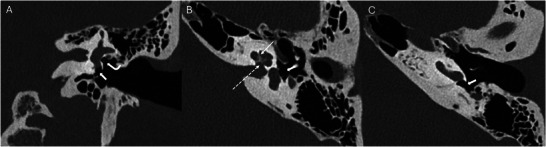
Photon‐counting detector computed tomography (PCD CT) images of the spiral structures within the human cochlea. Normal left temporal bone anatomic structures on PCD CT. A) Coronal PCD CT image demonstrating the position of the oval window (curved arrow) and round window (straight arrow). B) Axial PCD CT image at the level of the oval window (thick arrow) with insertion of the stapes crus. The modiolus (dashed arrow) and spiral lamina of the cochlear (thin solid arrow) can also be seen. C) At an inferior axial CT level, the round window is seen along the basal turn of the cochlea (arrow).
